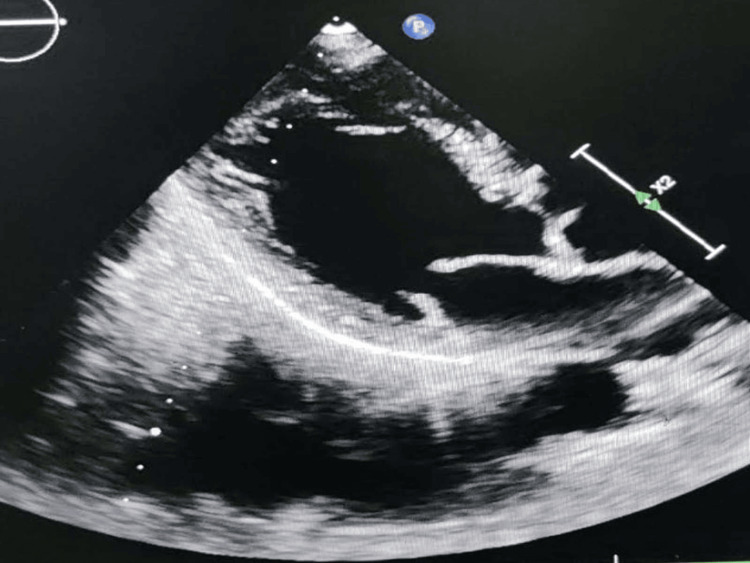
Parasternal long-axis view showing complete resolution of the ball valve thrombus with anticoagulation.
